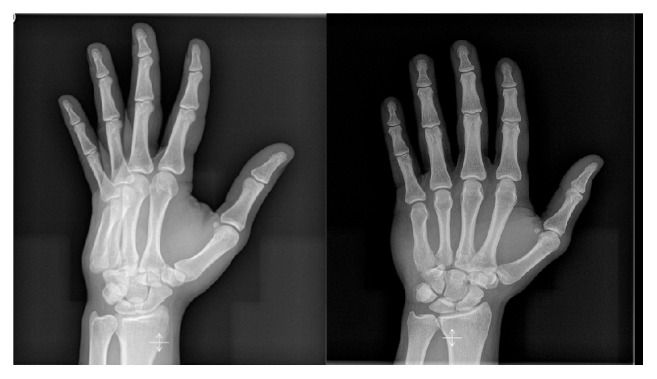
X-Ray: oblique and anteroposterior view of the hand. Oblique view shows a complete dislocation of the fourth and fifth CMC joints and a partial dislocation of the third CMC joint.
